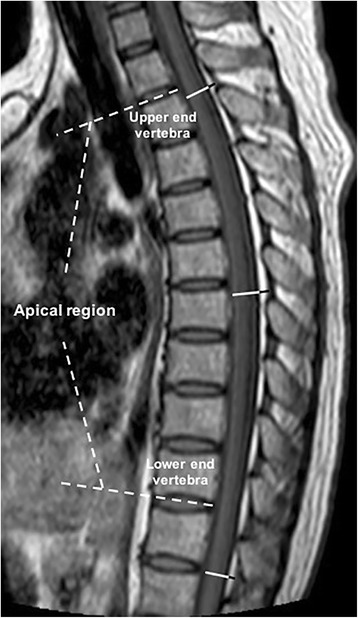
T1-weighted MR image of a normal control. The measurements of the dural sac (DS) are shown in white, and those of the epidural fat (EF) are shown in black. The measurements from proximal to distal level are EF_U_ and DS_U_, EF_A_ and DS_A_, and EF_L_ and DS_L_
